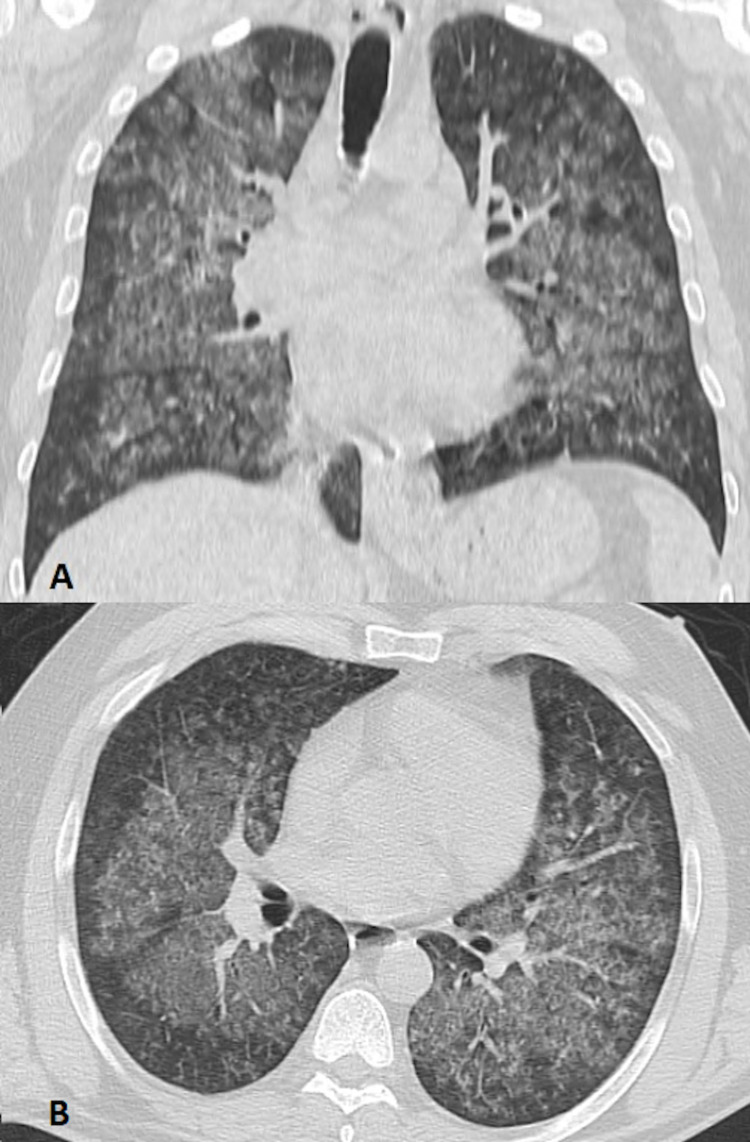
Coronal (A) and transverse (B) sections of CT of the chest with bilateral dense infiltrates on the day of admission
